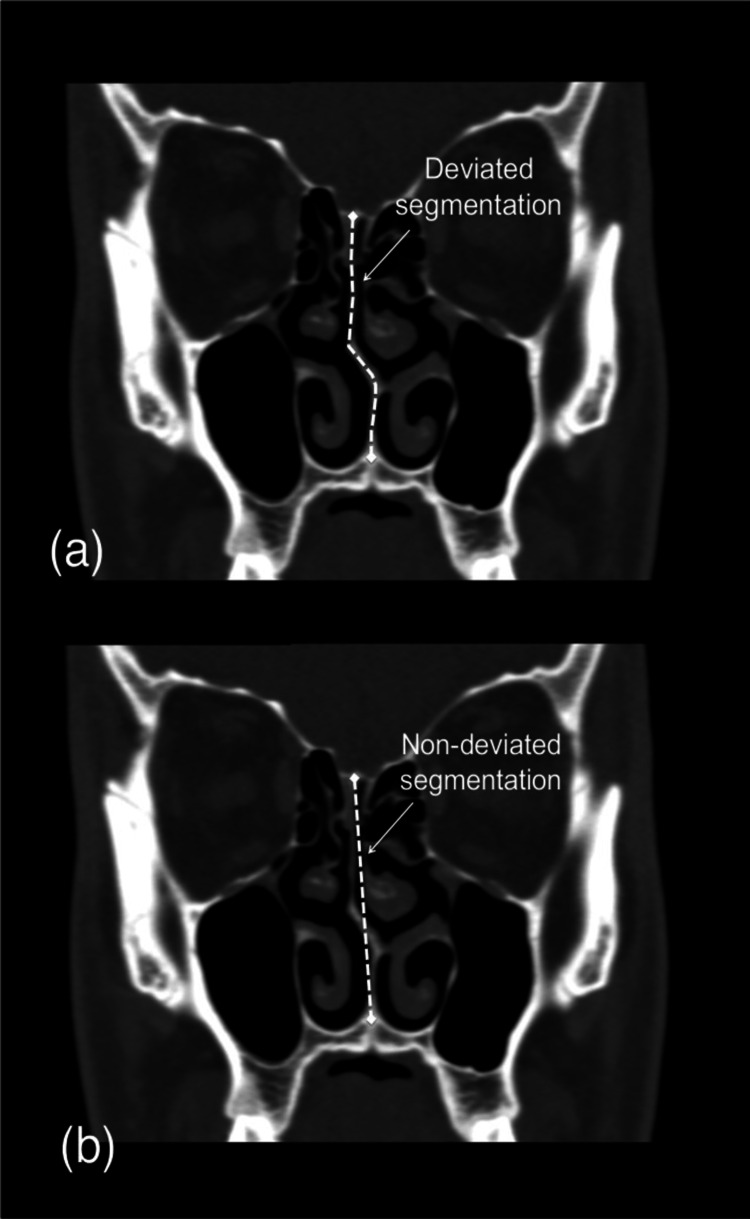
The CT scan of the nasal septum (a) Deviation of the nasal septum, (b) Normal nasal septum Image sourced from open access journal under a CC-BY license, contributed by Hartman et al. [6].
